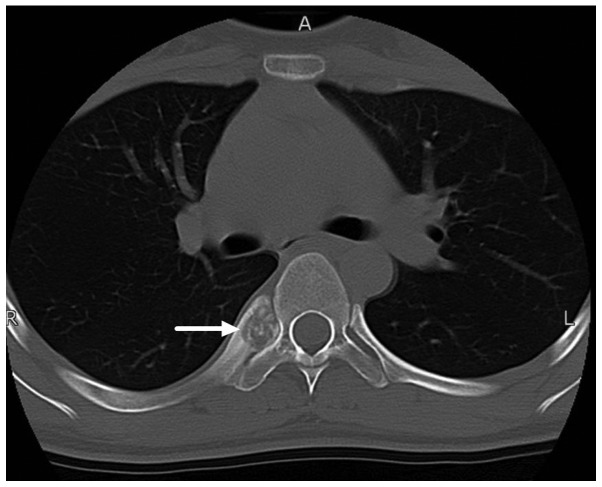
Computed tomography revealing the location of the lesion at the top of sixth right rib.
